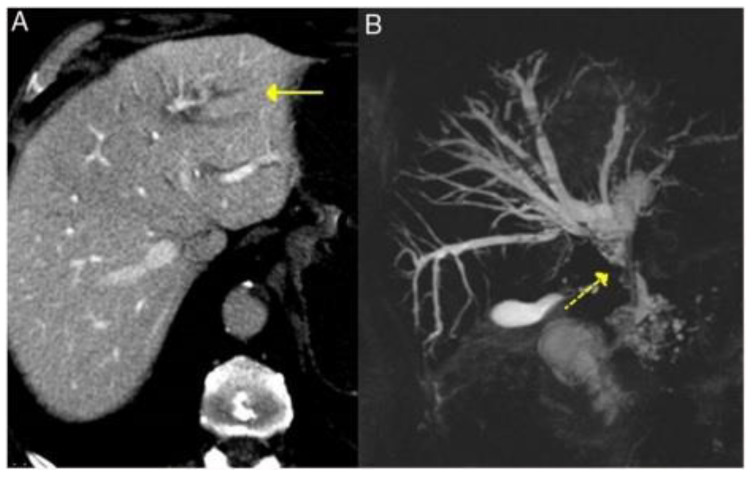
Typical features of primary sclerosing cholangitis in IgG4-RD seen on CT and MRI—dilatation of the intrahepatic bile ducts on the left side (solid arrow, A) and cholangio-MRI image showing dilatation of the extrahepatic and intrahepatic bile ducts (left and right) with areas of stenosis (dashed yellow arrow, B) [Adapted] [23].
